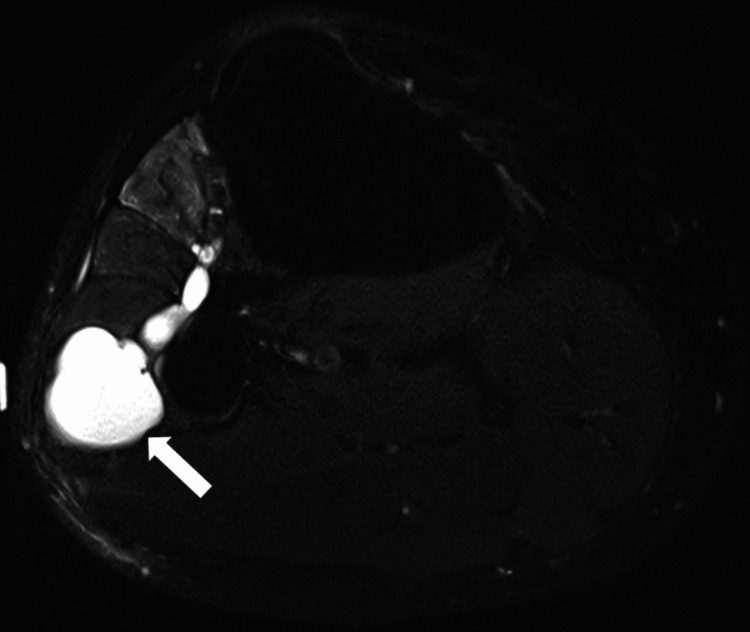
Postoperative MRI without contrast of the right lower extremity demonstrated the recurrent tubular intraneural ganglion cyst of the deep peroneal nerve and articular branch adjacent to the anterior aspect of the superior tibiofibular joint (white arrow)
